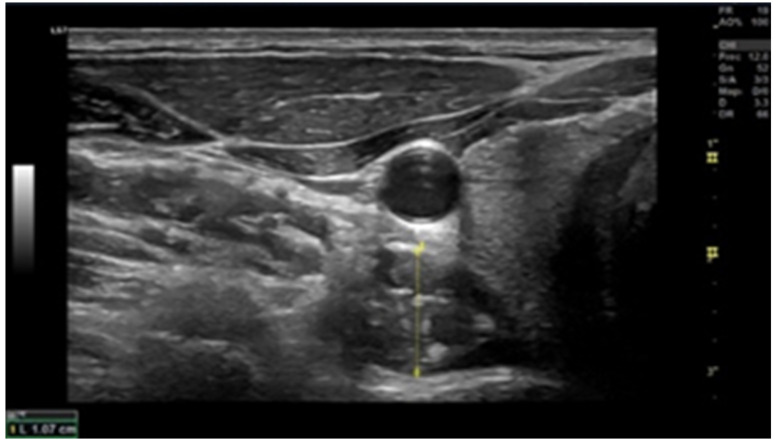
Longus colli ultrasound image at C6 (transverse view). The probe is placed on the midpoint of the ventral surface of the C6 vertebral body and the interface between the Lcol and the pre-fascial tissue surrounding the carotid artery.
